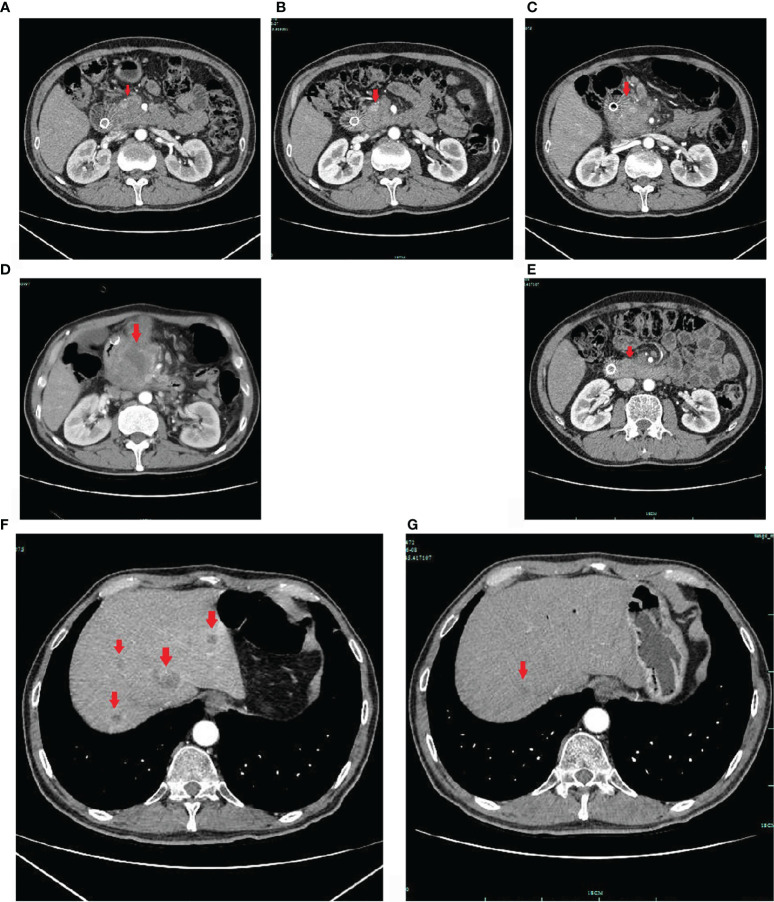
Enhanced CT images. The pancreatic mass indicated by the arrow on upper-abdomen enhanced CT took in June 2020 (A), in August 2020 (B), in October 2020 (C) and in November 2020 (D). The pancreatic cancer was responded to chemotherapy initially, but the disease was still progressed at later time. After sintilimab combined with S-1 treatment, the pancreatic lesion was significantly reduced in, and nearly disappeared in June 2021 (E). The hepatic metastases indicated by the arrows on upper-abdomen enhanced CT took in October 2020 (F), and in June 2021 (G). The hepatic metastasis was significantly reduced (G).
